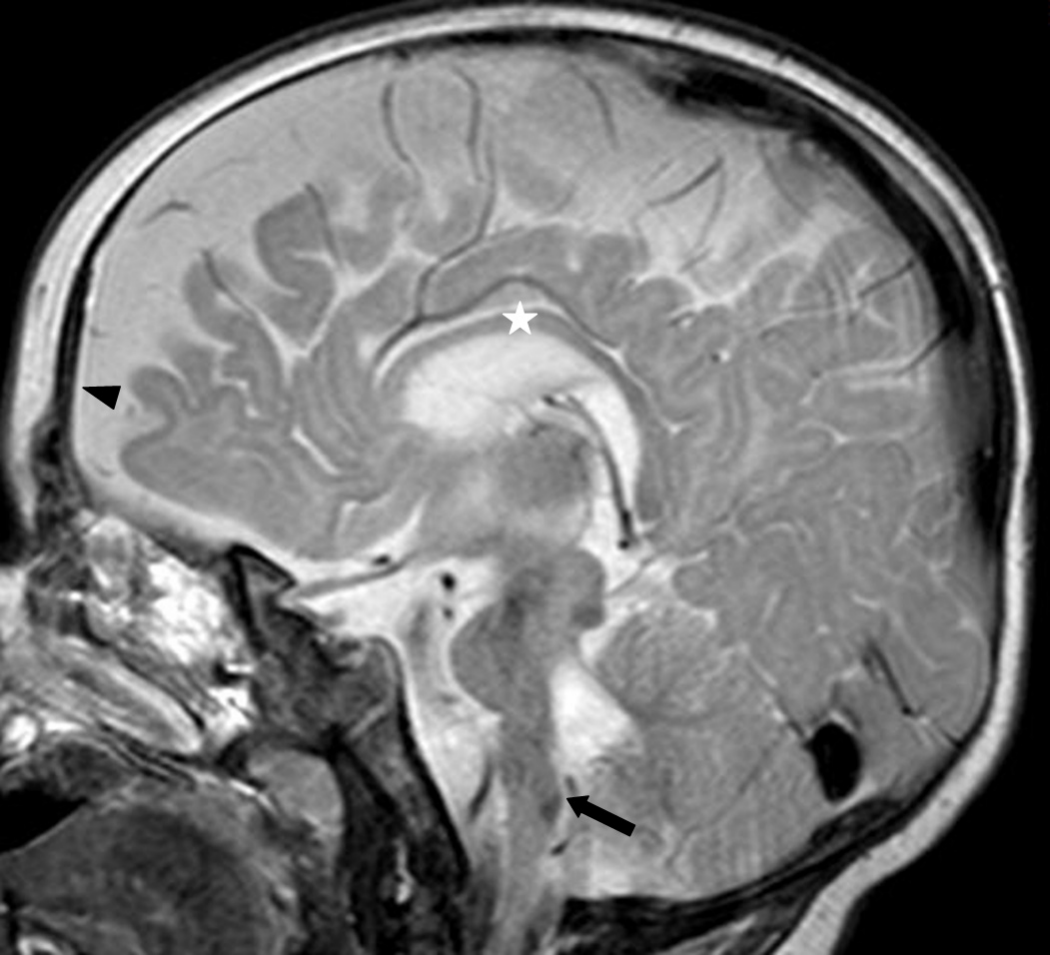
Sagittal magnetic resonance imaging scan of patient 4 at the age of 6 months. The frontal lobes are hypoplastic (arrowhead). The corpus callosum is thinned (star) and the brainstem is flattened (arrow).
